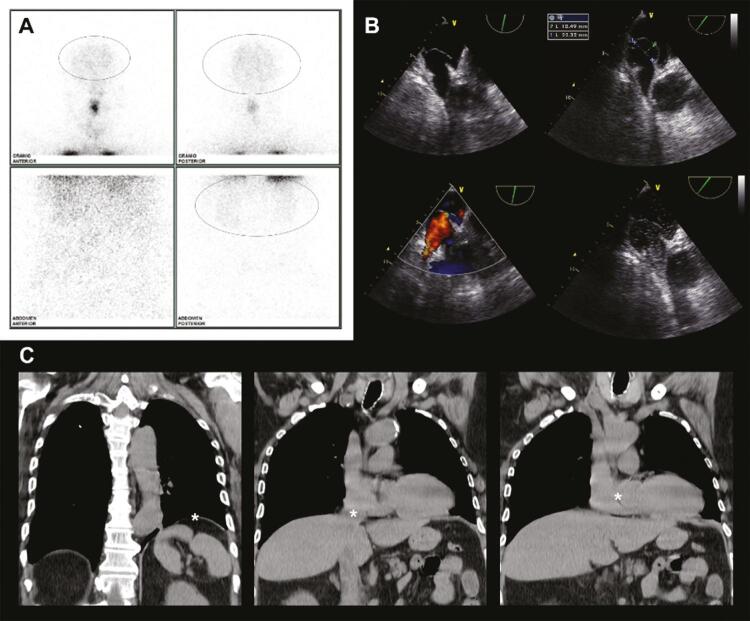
Painel A: Cintilografia de V/Q demonstrando captação, pelo cérebro e pelo rim, de macroagregado de albumina 99m Tc; Painel B: ETE demonstrando um aneurisma do septo interatrial e FOP, com um grande shunt D-E em repouso, visível por Doppler colorido e injeção de solução salina agitada; Painel C: Imagens de TC demonstrando a elevação do hemidiafragma esquerdo* alterando o eixo da veia cava inferior supra-hepática* e a posição do coração* horizontalmente.
